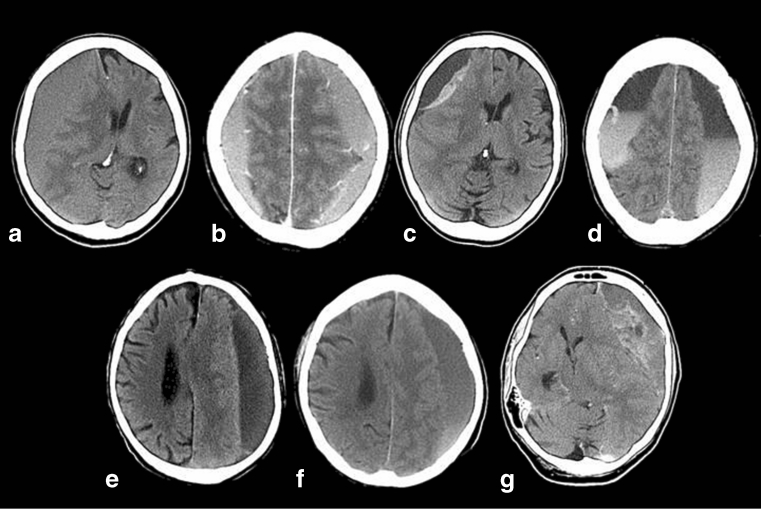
Representative CT densities of CSDHs according to the classification described by Nakaguchi et al. [17]. *Upper row*: densities of haematoma with high recurrence rate: a isodense; b hyperdense; c laminar; d separated. *Lower row*: densities of haematoma with low recurrence rate: e hypodense; f gradation; g trabecular
